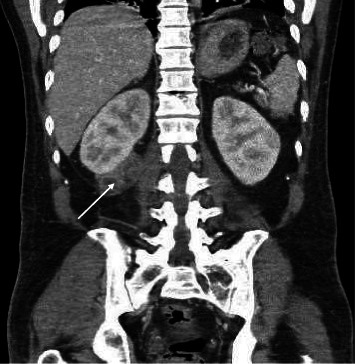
CT scan showing 4.9 cm cystic exophytic lesion in the anterior aspect of the inferior renal pole.
